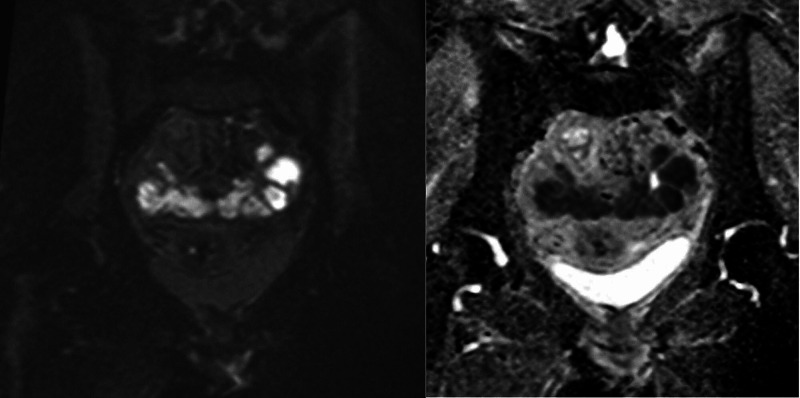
Coronal b800 diffusion weighted and apparent diffusion coefficient MRI pelvis showing high signal within the pelvic abscesses with signal drop out indicating restricted diffusion.
